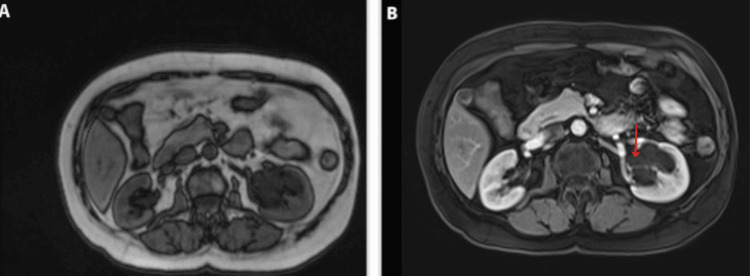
Left renal lymphangiectasia. MRI cystic image without left renal paracalyceal enhancement measuring 40x40x60 mm (transverse, cephalocaudal app). Axial section T1F (A), Axial T1 contrast-enhanced slice (B). Evidence of the pyelocaliceal system during the removal of contrast medium from the visualized cystic lesion, with no findings of extrinsic compression of the pyelocaliceal system.
